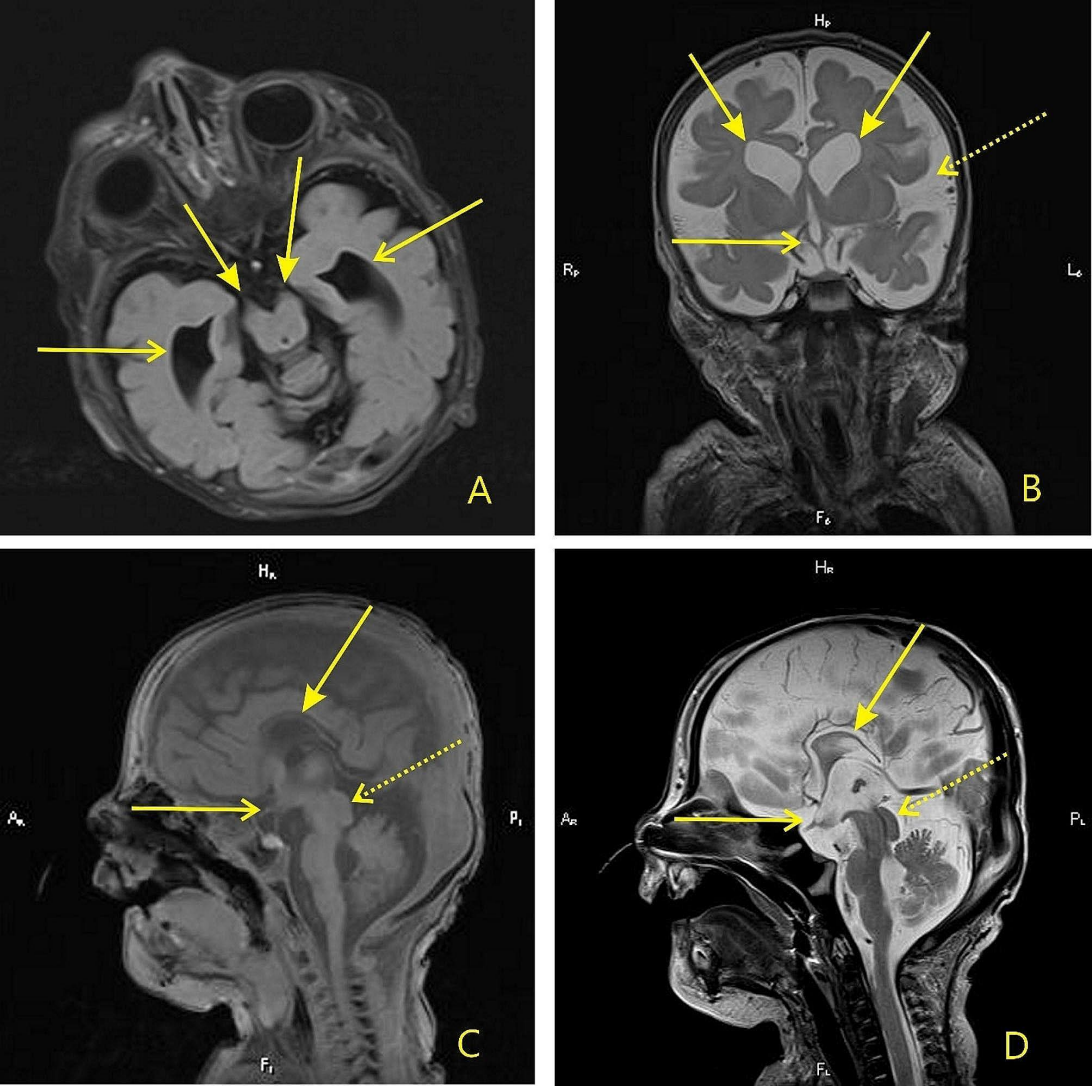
The results of brain MRI performed according to a routine protocol on a Magnetom Verio tomograph with 3T field induction and the use of a neonatal head coil, against the background of controlled sedation under the control of vital functions (SpO2, heart rate). (A): FLAIR image in the axial plane, arrows show the cerebral peduncles and brainstem reduced in size, open arrows show the enlarged temporal horns of the lateral ventricles; (B): T2WI in the frontal plane, arrows show dilated bodies of the lateral ventricles, the chiasm is hypoplastic (shown by an open arrow), dilated external cerebrospinal fluid spaces (dotted arrow); (C): T1WI in the sagittal plane, the arrow shows the hypoplastic corpus callosum, the genu is not developed, the splenium is absent, the hypoplastic chiasm is shown by an open arrow, the enlarged quadrigeminal plate is shown by a dotted arrow. (D): T2WI in the sagittal plane, the arrow shows the hypoplastic corpus callosum, the genu is not developed, the splenium is absent, the hypoplastic chiasm is shown by an open arrow, the enlarged quadrigeminal plate is shown by a dotted arrow
